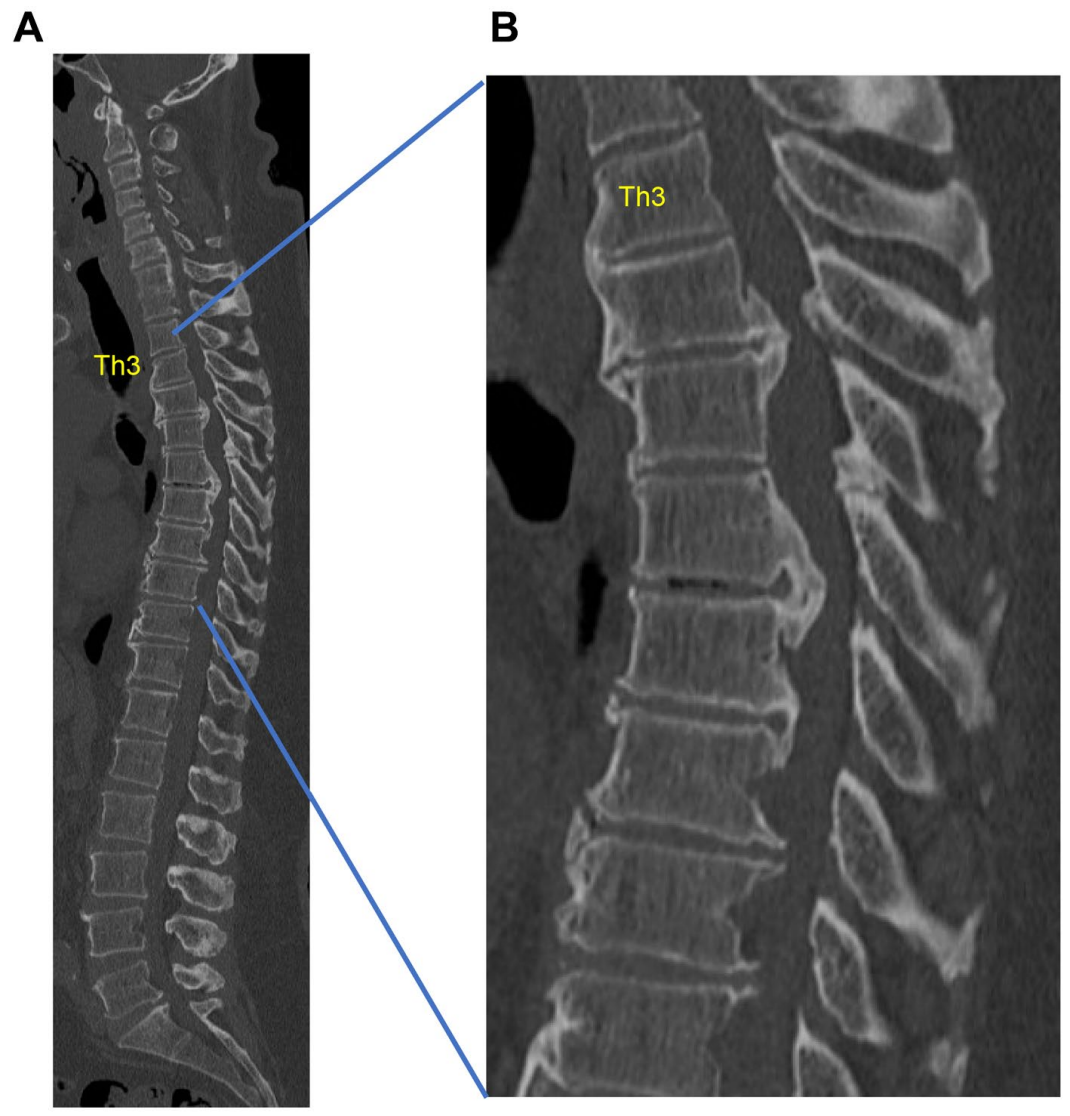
Example of thoracic ossification of the posterior longitudinal ligament (OPLL) in a 53-year-old obese male with type 2 diabetes mellitus. The spinal canal is markedly narrowed by thoracic OPLL, with inability to walk. (A) Sagittal computed tomography (CT) of the whole spine. (B) Sagittal CT of the thoracic spine. This is a case from our institution.
